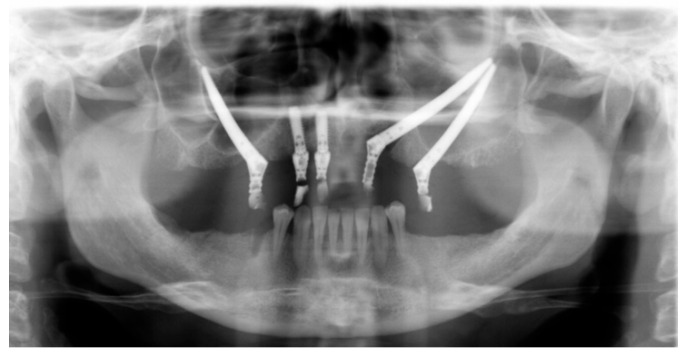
Panoramic radiograph, showing two ZIs on the left side with their apex in close contact without adequate inter-implant distance.
